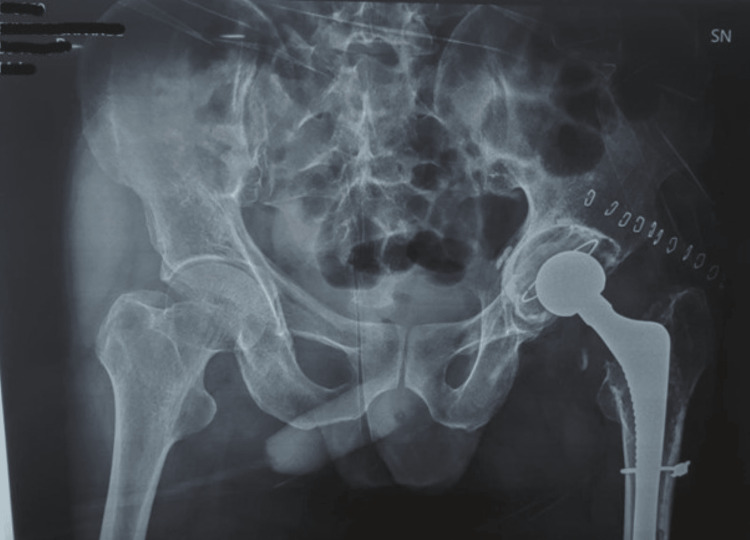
Postoperative plain radiograph of the pelvis with both hips
